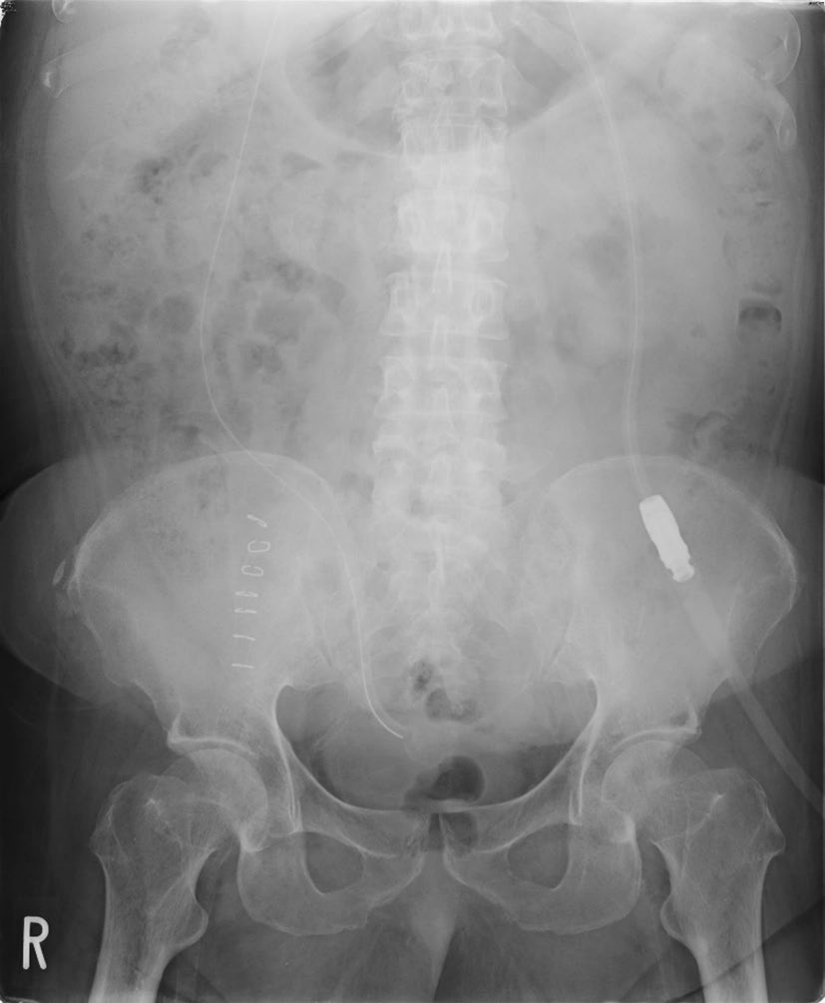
Abdomen X-ray after insertion of PD catheter
